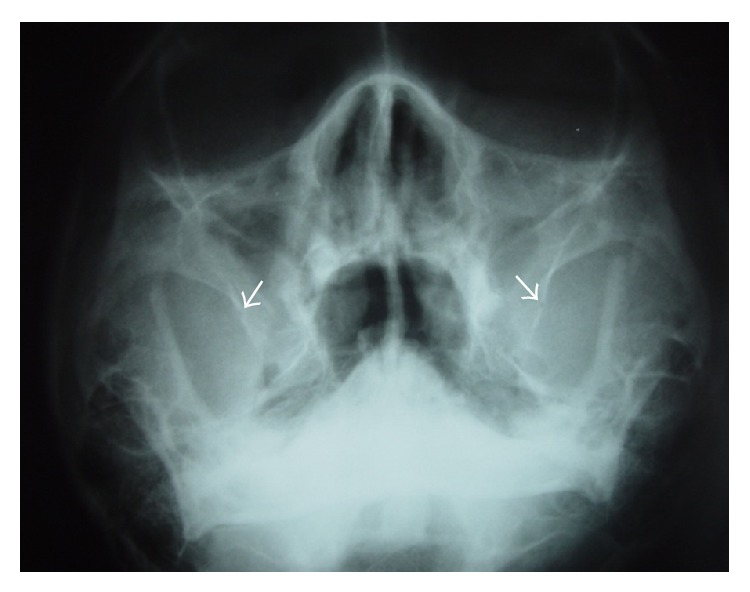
Posteroanterior radiograph of the maxillary sinus (Water's projection) showing a radiolucent area corresponding to the route of the neurovascular bundle (white arrows).
